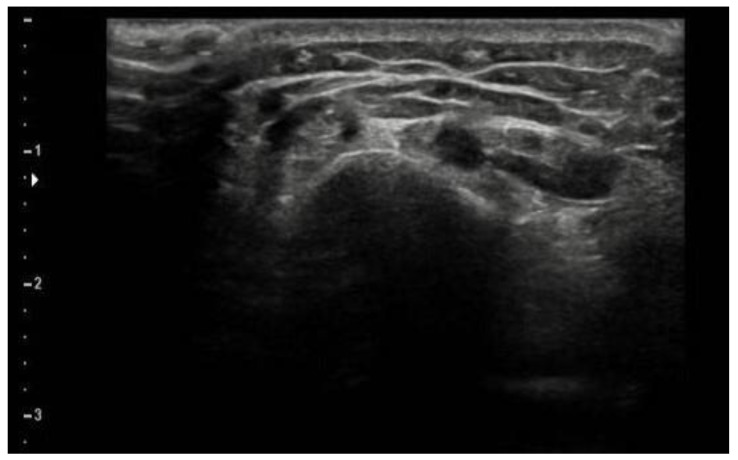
Doppler ultrasound of the wrist: thickening of the subcutaneous soft tissues associated with a minimal flap of effusion in accordance with the tendon sheath of the extensor tendons of the fingers is seen. No further alterations that can be evaluated with this method are observed.
